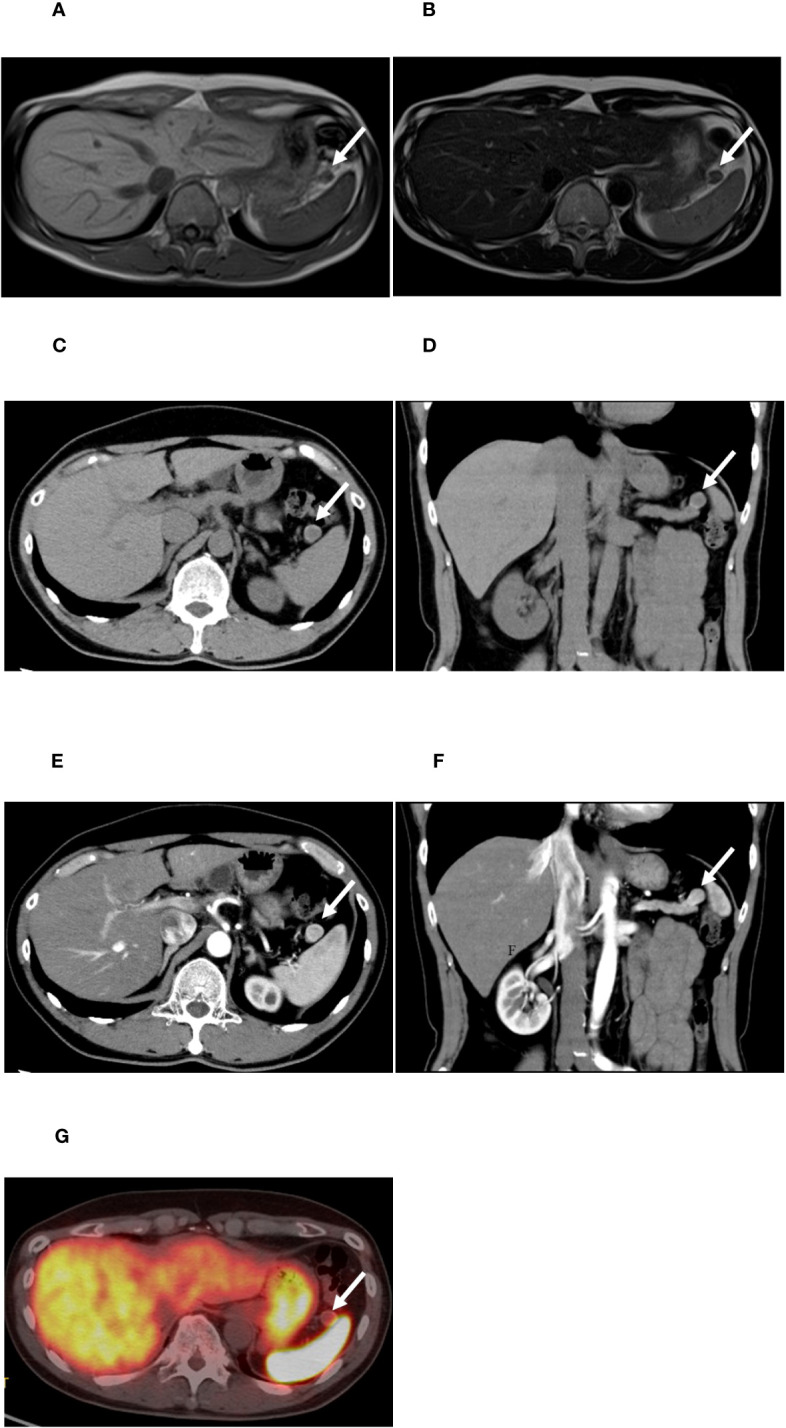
Images obtained by conventional clinical examinations. (A, B) Magnetic resonance imaging of the abdomen. (A) T1-weighted image. (B) T2-weighted image. (C–F) Computed tomography (CT) scan of the nodule of the pancreatic tail. (C, D) Plain CT; (E, F) early phase of contrast-enhanced CT. (G)
